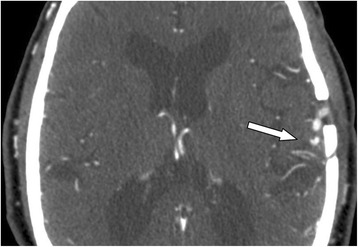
CT-angiography demonstrating the patency of the by-pass graft. CT-angiography after EC-IC bypass shows left craniotomy defect with pronounced distal MCA flow from the left superior temporal artery (*arrow*)
